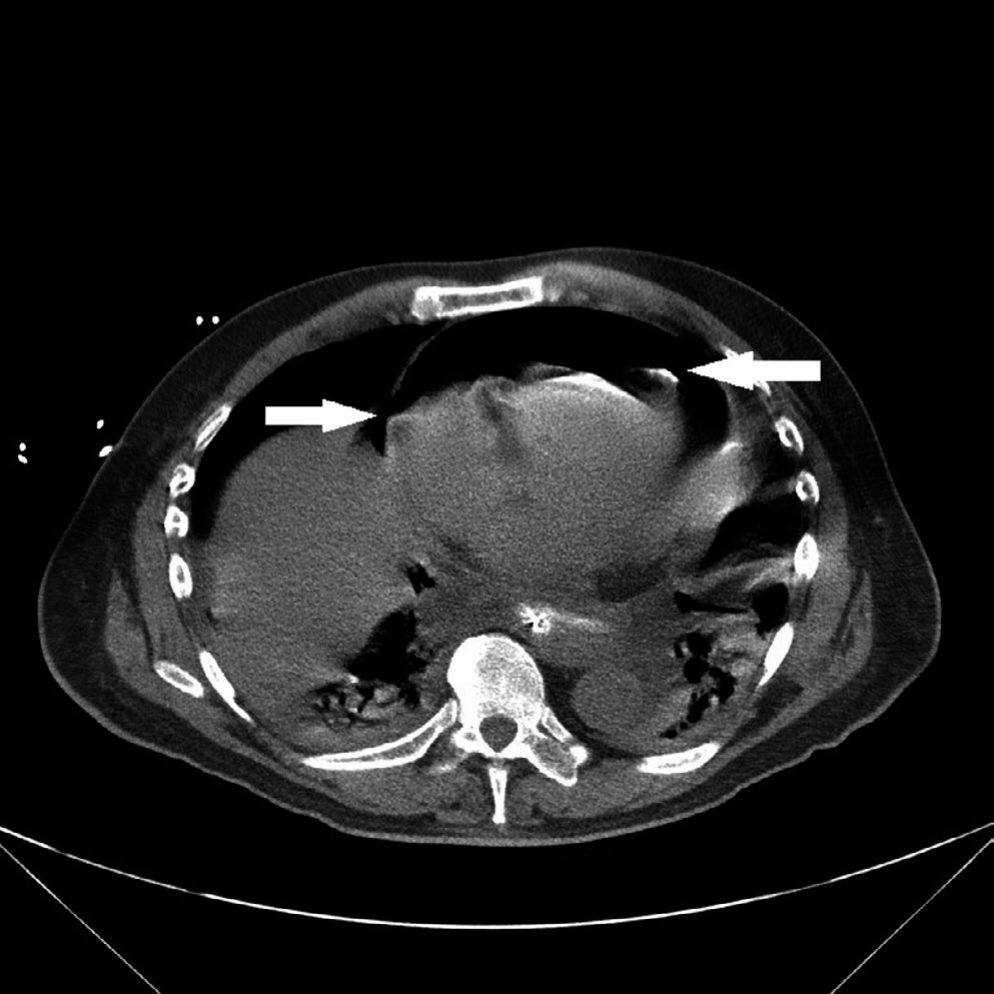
Computed tomography scan which shows dependent air‐fluid levels in the pericardial sac (white arrows)
